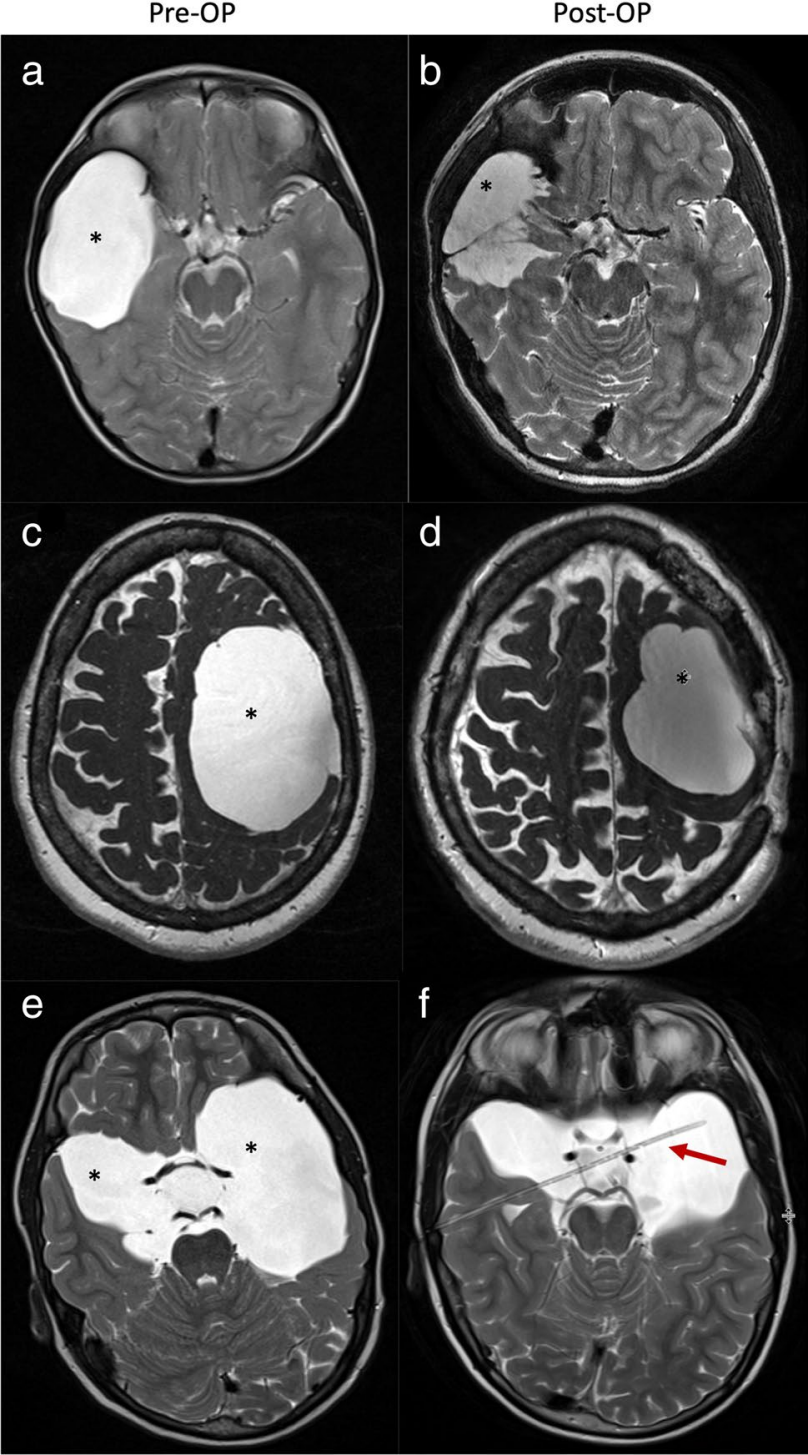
MR imaging of patients with cerebral arachnoid cysts (*) before (left) and after (right) microsurgical/endoscopic cyst wall fenestration (images a + b and c + d) and catheter implantation (images e + f) (red arrow). Volume reduction was achieved with all surgical approaches
